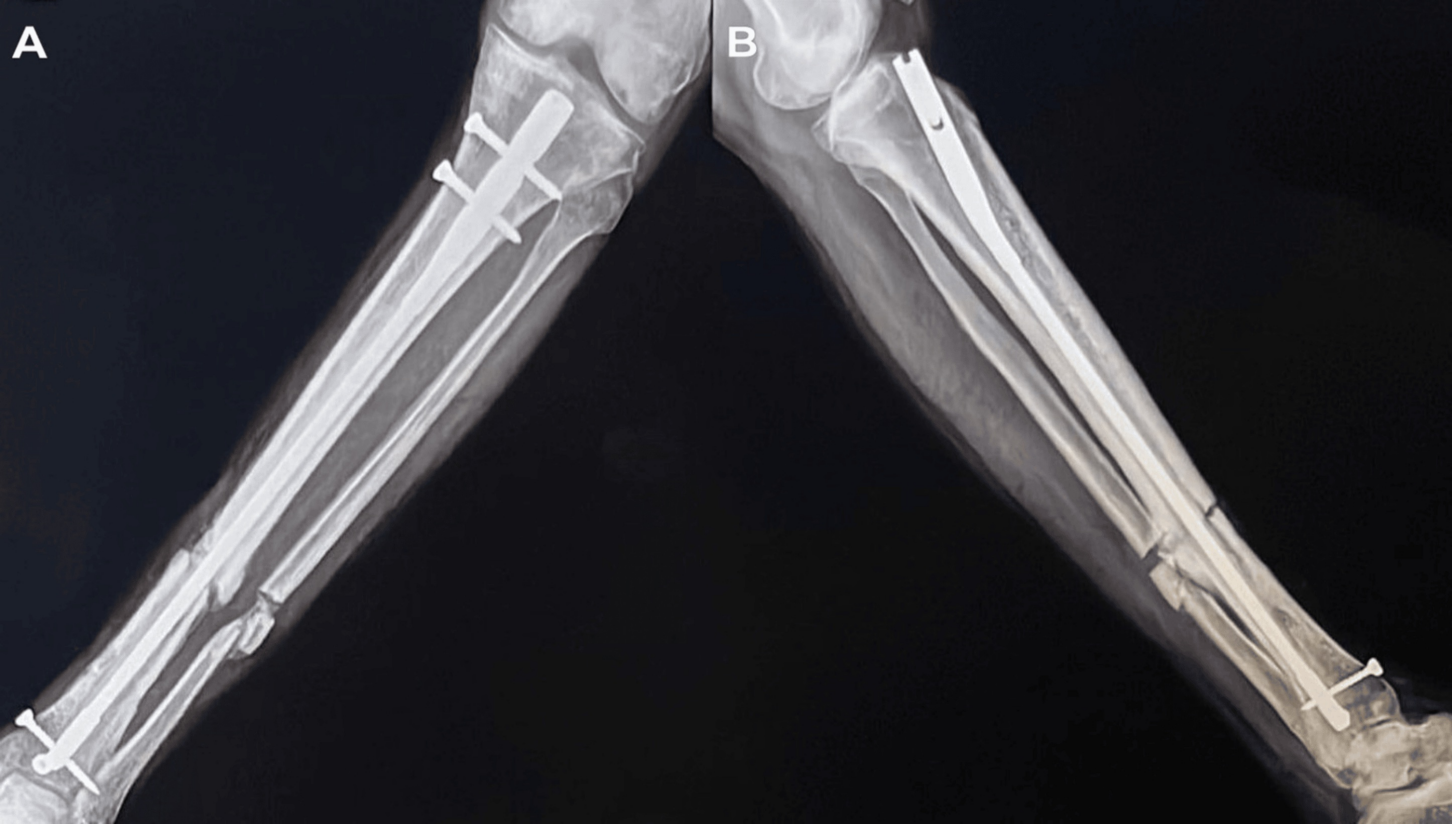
A-B) Left tibia and fibula AP and lateral X-ray views with signs of fracture union at two months post-solid intramedullary nailing. AP: anterior posterior
